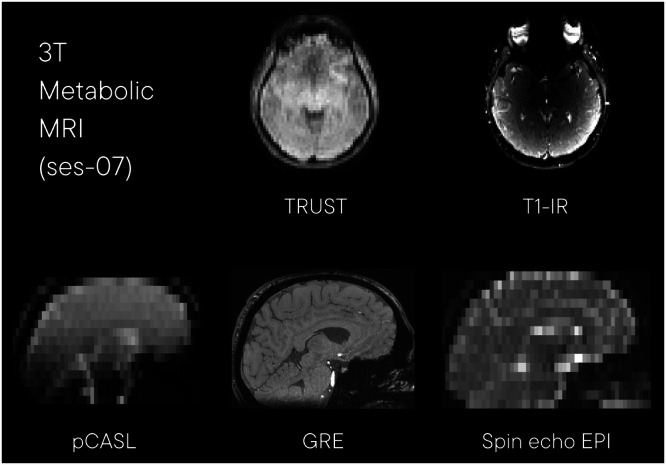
Example images from session 07, 3 T functional and perfusion MRI. TRUST: T2 relaxation under spin tagging; T1-IR: T1 inversion recovery; pCASL: pseudo-continuous arterial spin labelling; GRE: Single-echo 3D gradient echo sequence; EPI: echo planar image.
